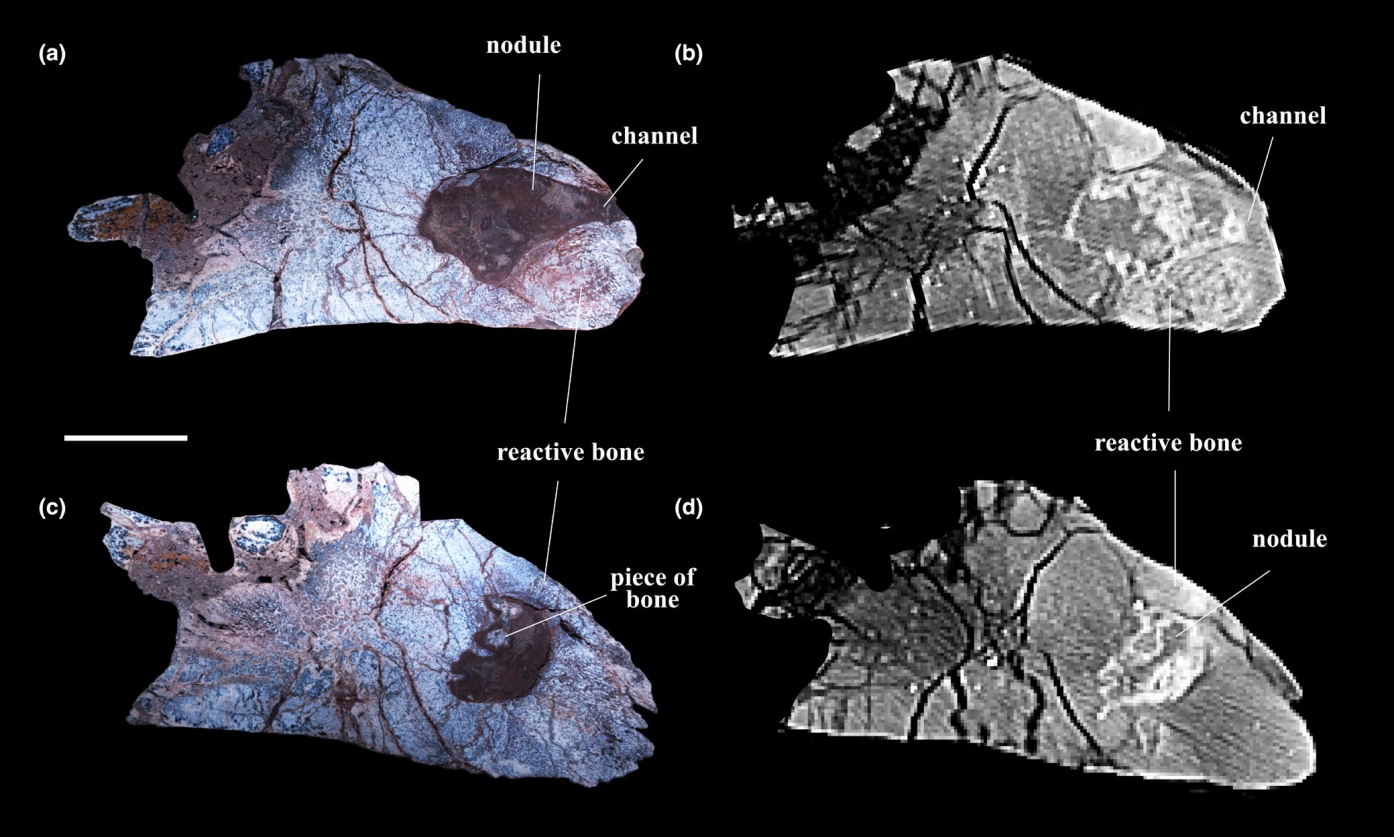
(a) and (c) Cross‐sections of the respective pathological regions, P3 and P2 (as indicated in Figure 1c); (b) and (d), the CT scan images of the pathological regions on the posterior side of PN14‐108. Scale bar equals 20 mm.
